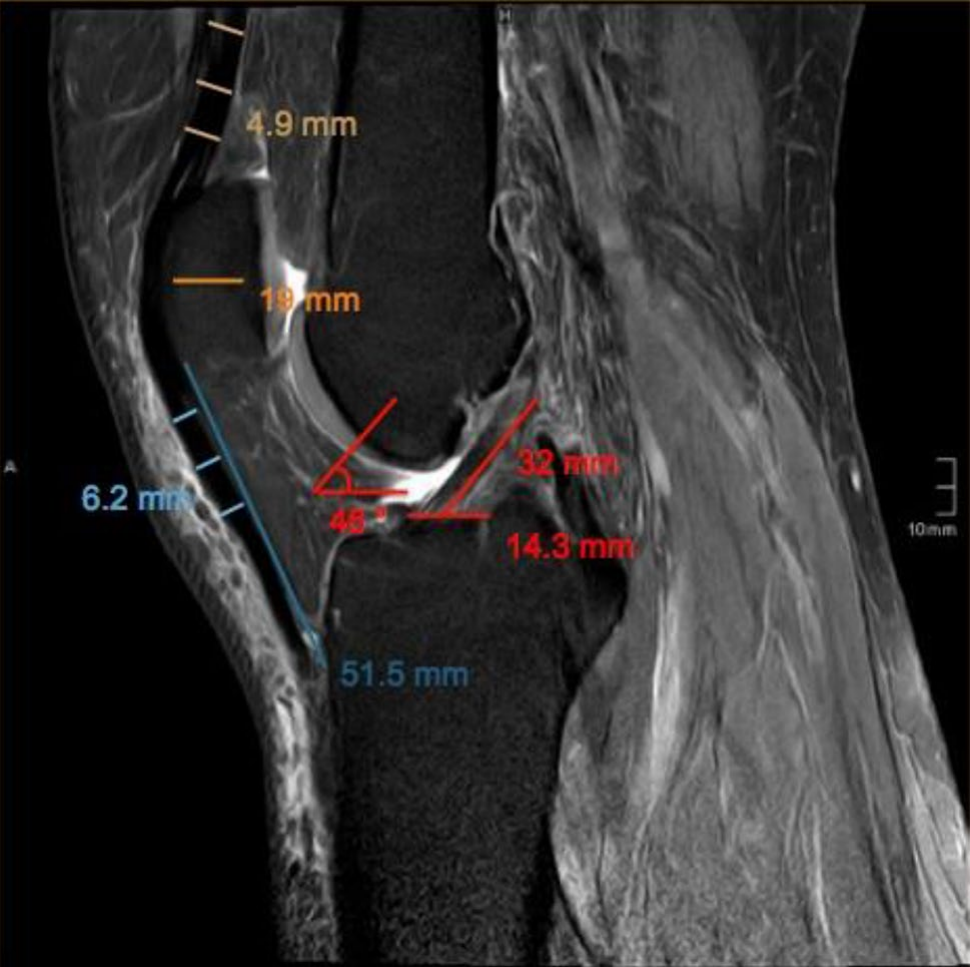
Measurements of the thickness of the quadriceps tendon (QT), patella, length of the patella tendon (PT), thickness of the patella tendon, length and angle of the anterior cruciate ligament (ACL) and tibial insertion site of the ACL on a sagittal MRI (T2 sequence)
